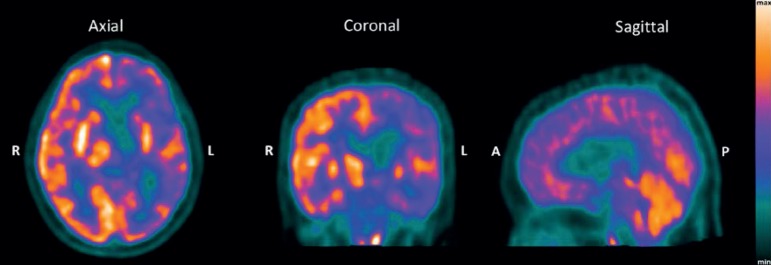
Positron emission tomography (PET) with ^18^F-FDG. Note
